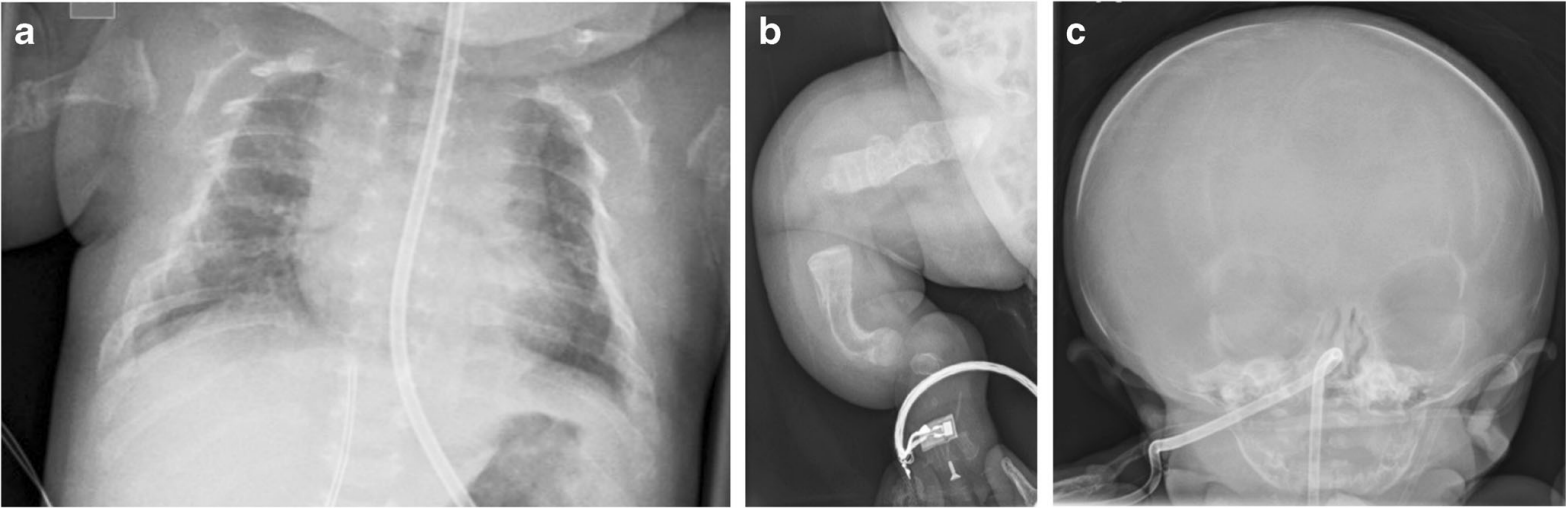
Radiographic features of a 2-week-old infant of unknown gender with severe osteogenesis imperfecta type III. Anteroposterior radiographs show broad ribs with multiple fractures (‘‘beading”) in the chest (a), broad tubular bones with multiple fractures and bowing of the tibia and fibula of the right lower limb (b), and deficient ossification of the skull vault (c; less severe than in the infant shown in Fig. 14)
